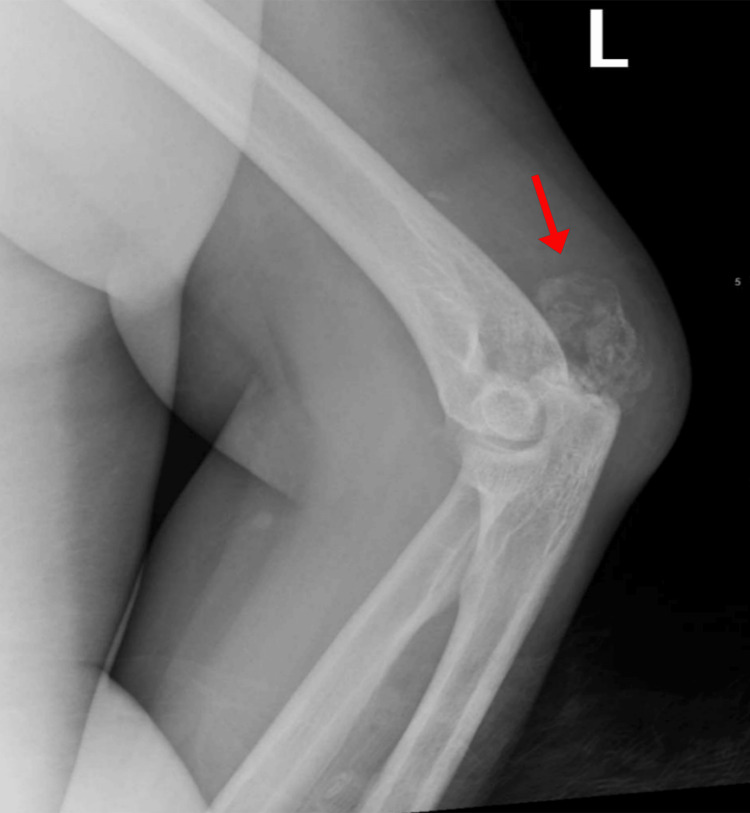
X-ray of the left elbow demonstrating periarticular calcium-phosphate deposits.
